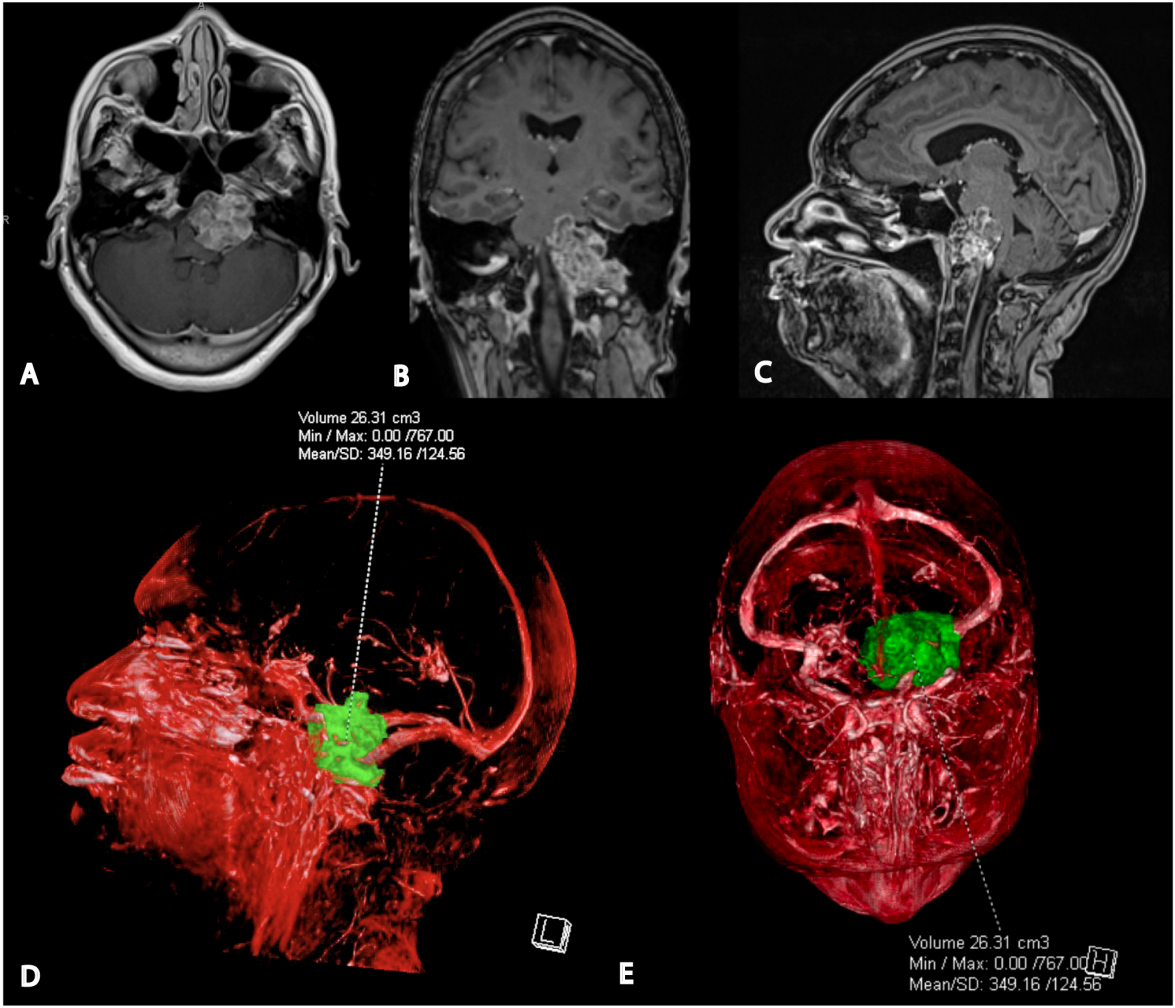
Preoperative magnetic resonance imaging and 3D tumor segmentation. (A) Axial T1-weighted post-gadolinium MRI shows the tumor’s proximity to the left vertebral artery, proximal basilar artery, and internal carotid artery. (B) Coronal T1-weighted post-gadolinium MRI indicates the involvement of the left occipital condyle, jugular foramen, and hypoglossal canal. (C) Sagittal post-gadolinium T1 weighted MRI demonstrating significant mass effect on pons and medulla. (D) Lateral view of the 3D segmented tumor volume from the patient’s left side. (E) Superior view of the 3D segmented tumor volume.
